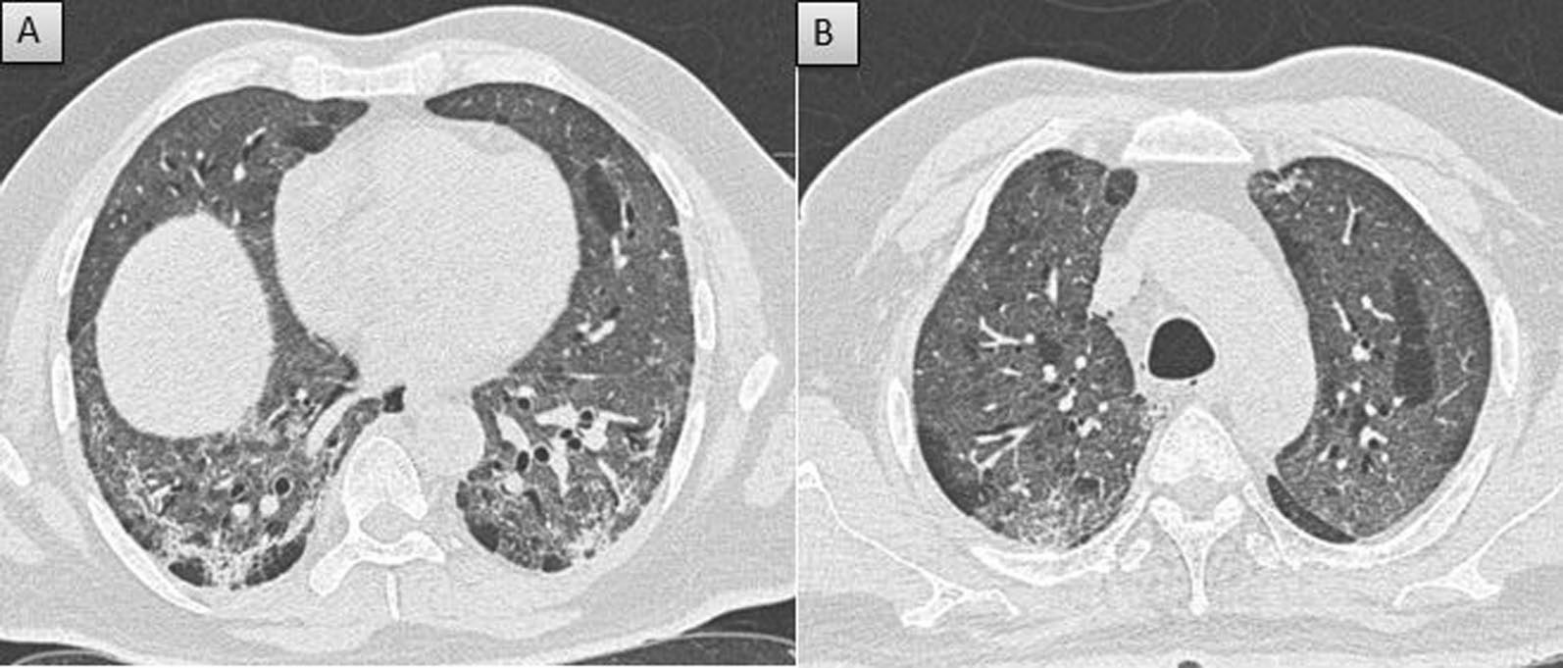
Severe severity in non-vaccinated patient: A and B Axial CT thorax (lung window) of a 57-year-old non-vaccinated COVID pneumonia patient shows diffuse confluent ground glass opacities with CT severity score of 22/25 (severe severity)
